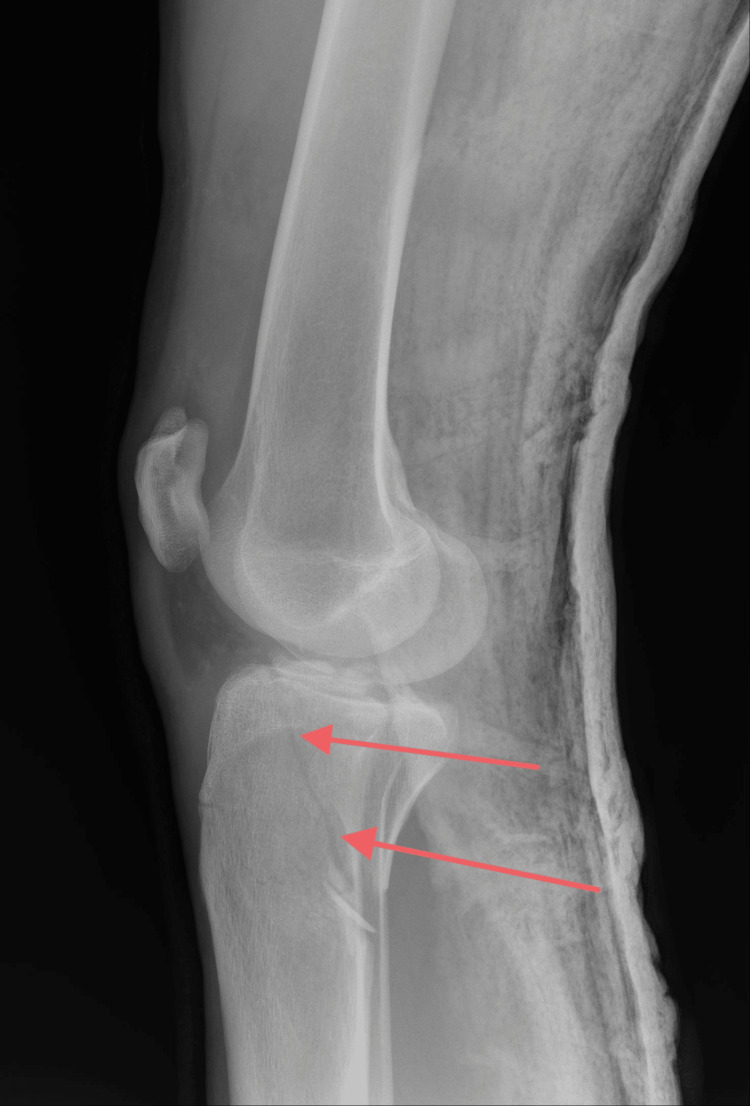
X-ray (Lateral view) showing a Schatzker type V fracture Red arrows point to the fracture line.
